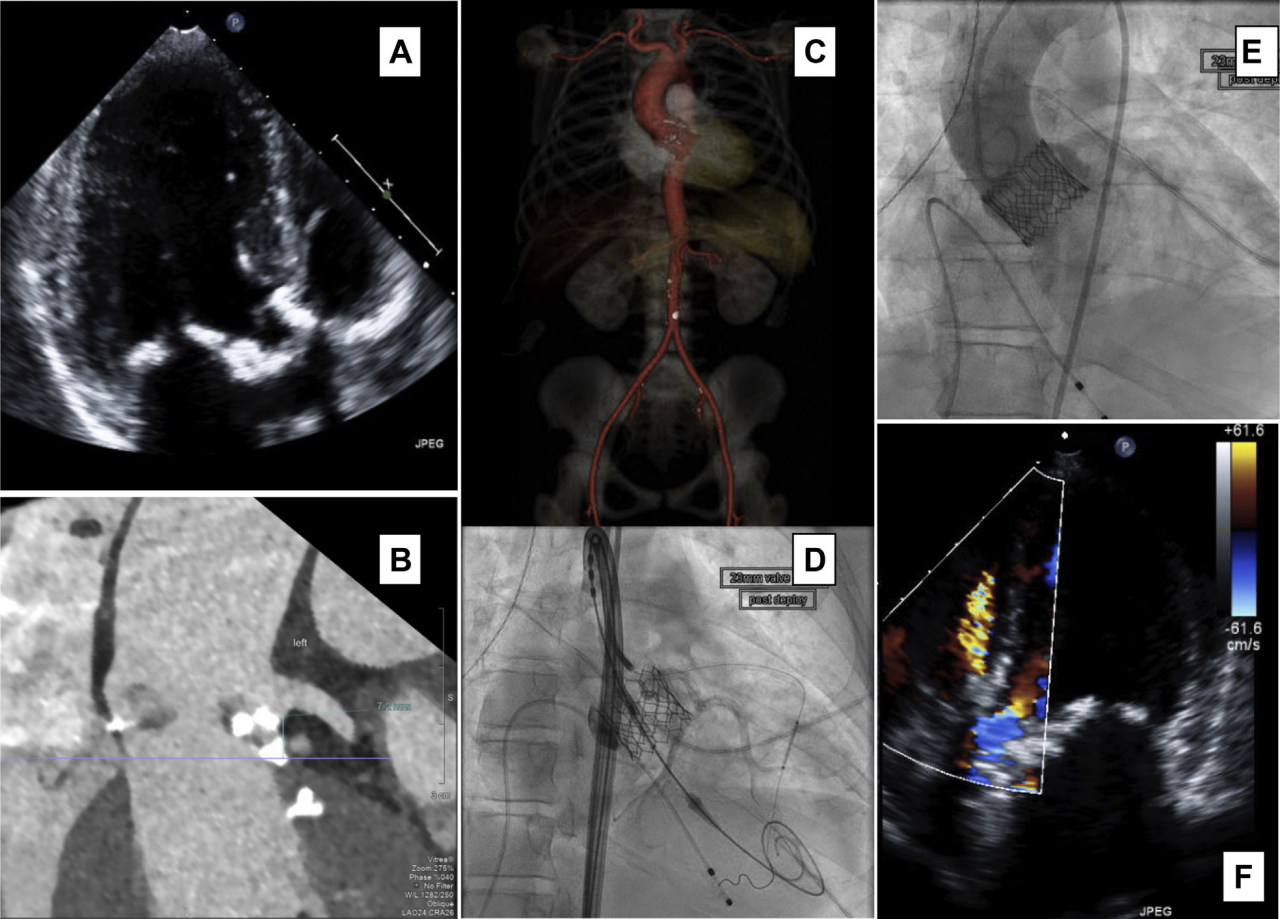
Multimodality Imaging Used for Procedural Planning (A) Calcification of the mitral and aortic valves on echocardiography. (B) On gated cardiac computed tomography, short left main coronary ostial height and calcific nodule in the left ventricular outflow tract were appreciated. (C) Computed tomographic reconstruction of aorta and iliofemoral vessels. (D) Nonselective left coronary injection after valve deployment showing patent left main coronary artery and undeployed coronary stent in the left anterior descending coronary artery. (E) Final aortic root angiography. (F) Post-procedural echocardiography, with normal gradients and no significant paravalvular leak but suggestion of a fistula between the aorta and right ventricular outflow tract.
